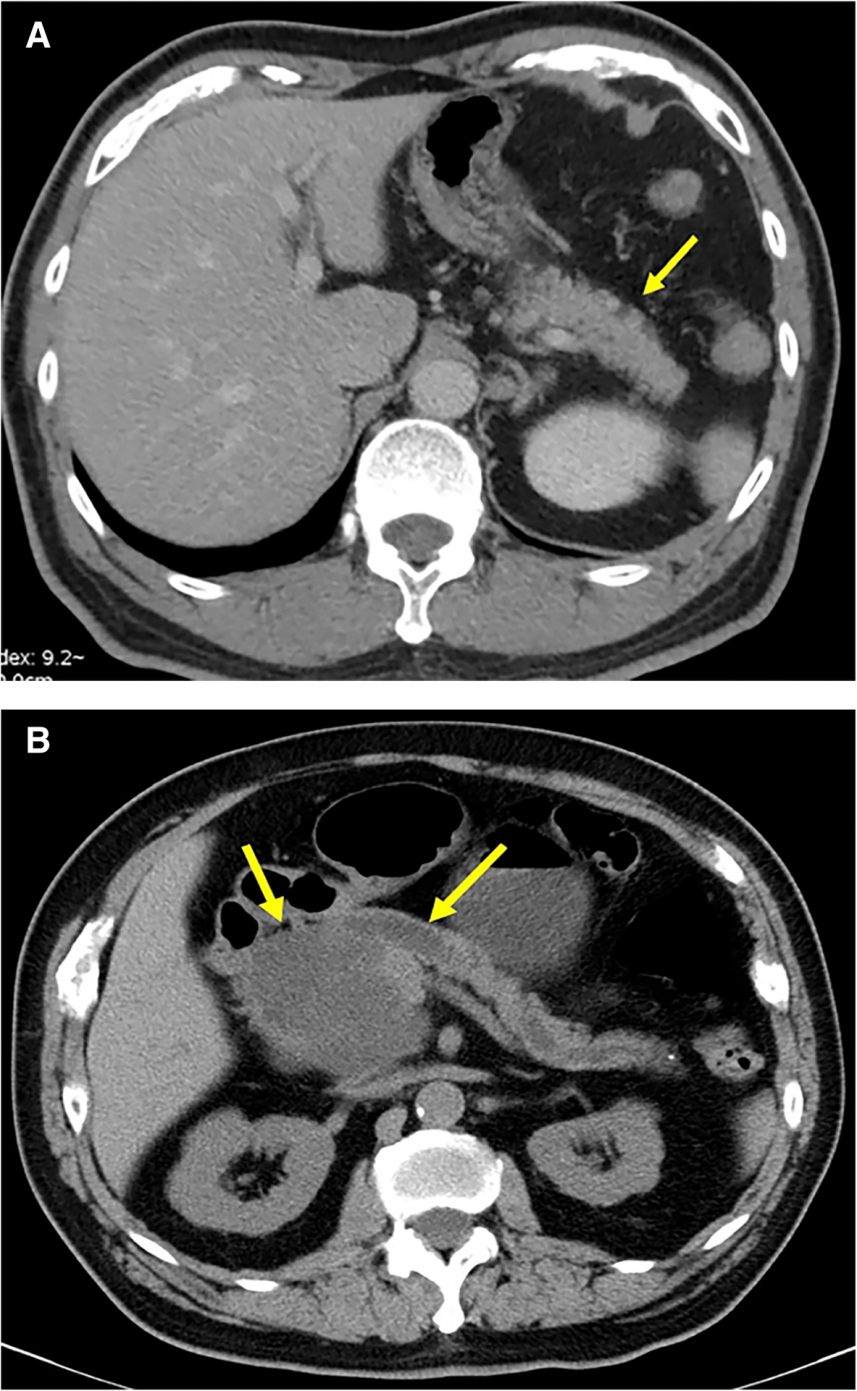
Imaging features of PCLs detected by CT. a a patient with a small (6 mm) cyst in the body of pancreas. Transverse image from contrast-enhanced CT scan shows slightly low-density lesion (arrow) in the body of the pancreas and is easy to be misdiagnosed as pancreatic fat infiltration. b a patient with a cyst in the head of pancreas. Transverse image shows a low-density lesion in the head of pancreas with dilation of main pancreatic duct (arrow)
